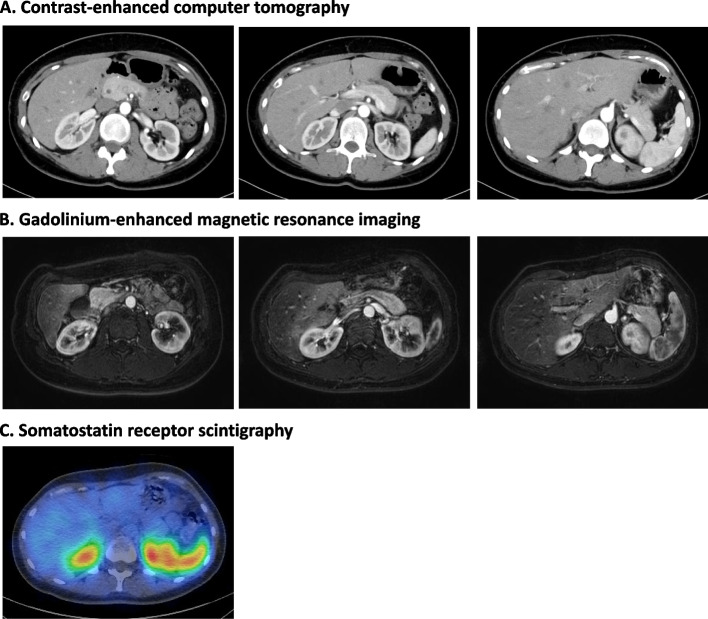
Imaging analyses of the pancreas of the case. A Contrast-enhanced computer tomography. B Gadolinium-enhanced magnetic resonance imaging. C Somatostatin receptor scintigraphy
